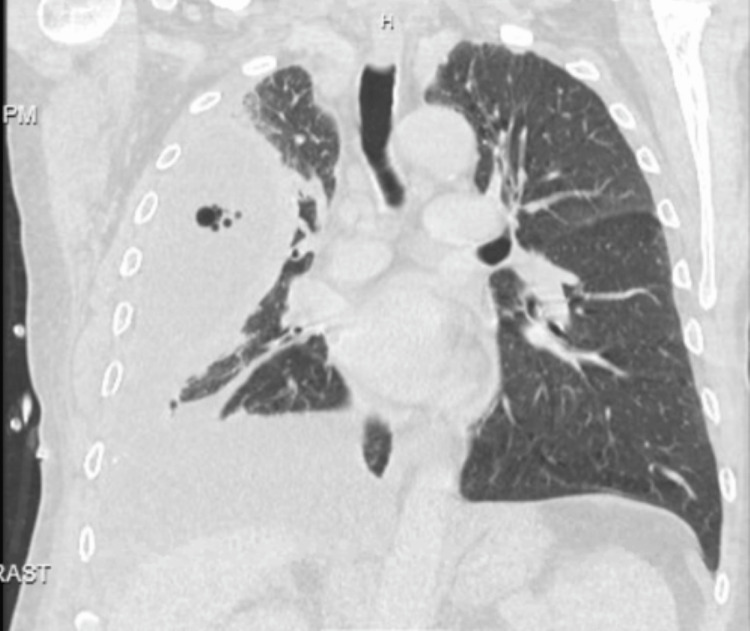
CT chest. Coronal view Showing a large, loculated right pleural effusion with the right chest drain in the appropriate position. Air pockets in the fluid could be related to the presence of the chest drain or could represent an infectious process. There is dense compressive atelectasis of the right lung and moderate left pleural effusion.
